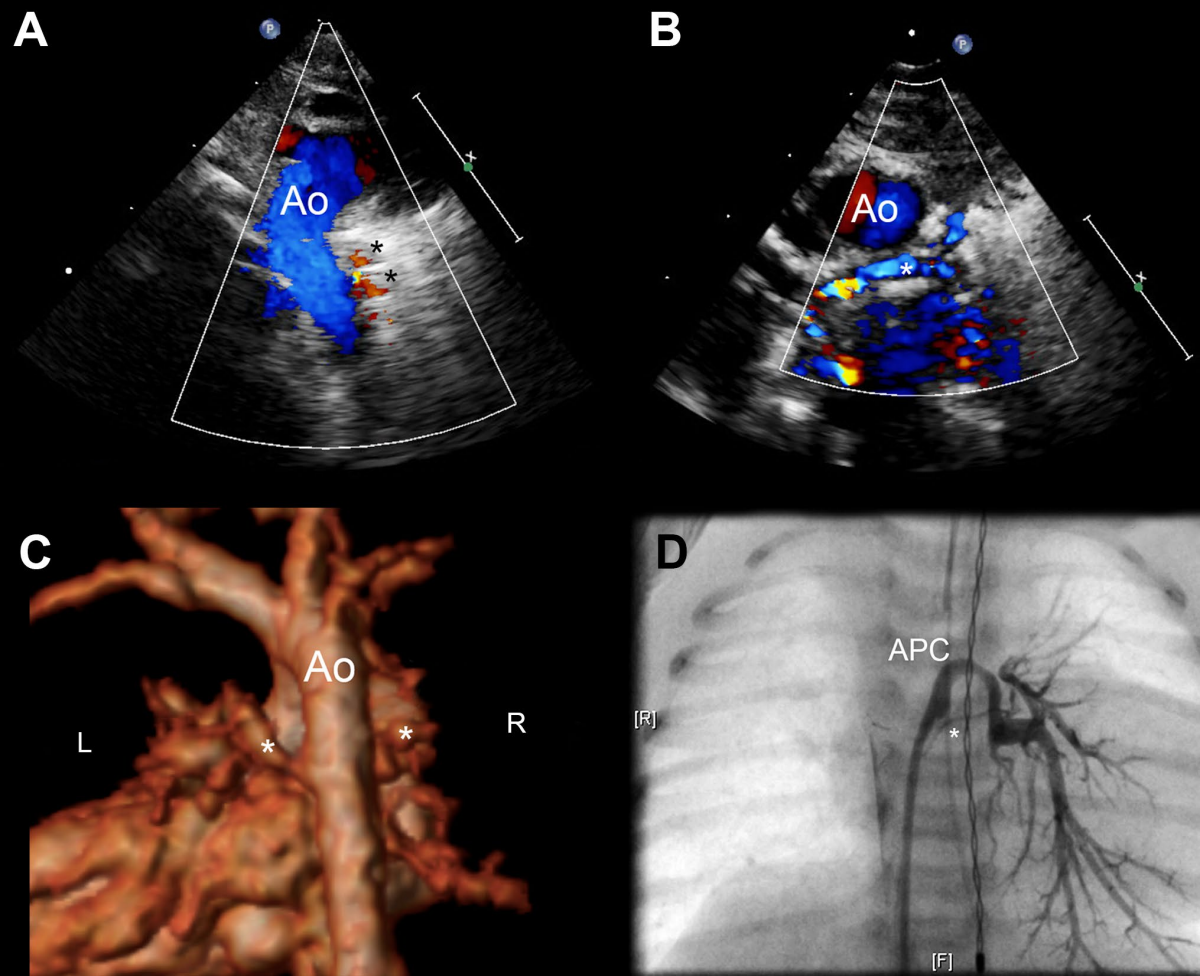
a Echocardiography immediately after birth in a patient antenatally diagnosed with pulmonary atresia and ventricular septal defect showing a right sided aortic arch and collateral vessels (asterisked) arising from the descending aorta. *Ao* aorta. b Parasternal short axis view from the same study showing suspected confluent, but severely hypoplastic pulmonary arteries (asterisked). *Ao* aorta. c 3D reconstructed MRI on day 1 of life in the same patient, clearly demonstrating large collaterals to the left and right lung from the descending aorta. The possibility of a small native left pulmonary artery was raised but the study was not conclusive. CT imaging was subsequently performed at 7 days—no native pulmonary arteries were identified. d Still from aortic injection during cardiac catheterisation on day 13 of life. Tiny confluent branch pulmonary arteries were identified (asterisked), in addition to the major collaterals previous described
